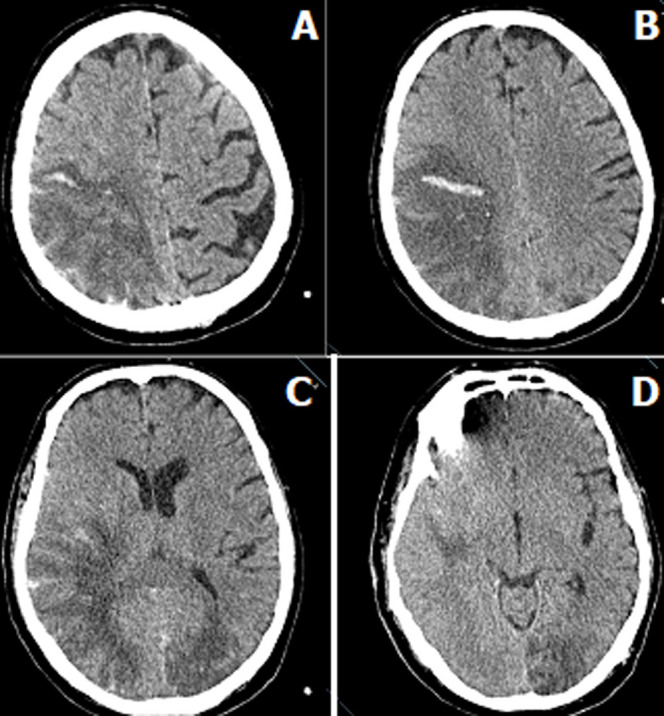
cranial computed tomography; A) hypodense areas in the frontal, parietal and temporal lobes on the right, B) occipital lobes, erasing of the cortical sulci due to edema in these areas, C) compression of the posterior horn of the right ventricle, D) hyperdense foci compatible with bilateral hemorrhagic changes accompanying the hypodense areas
